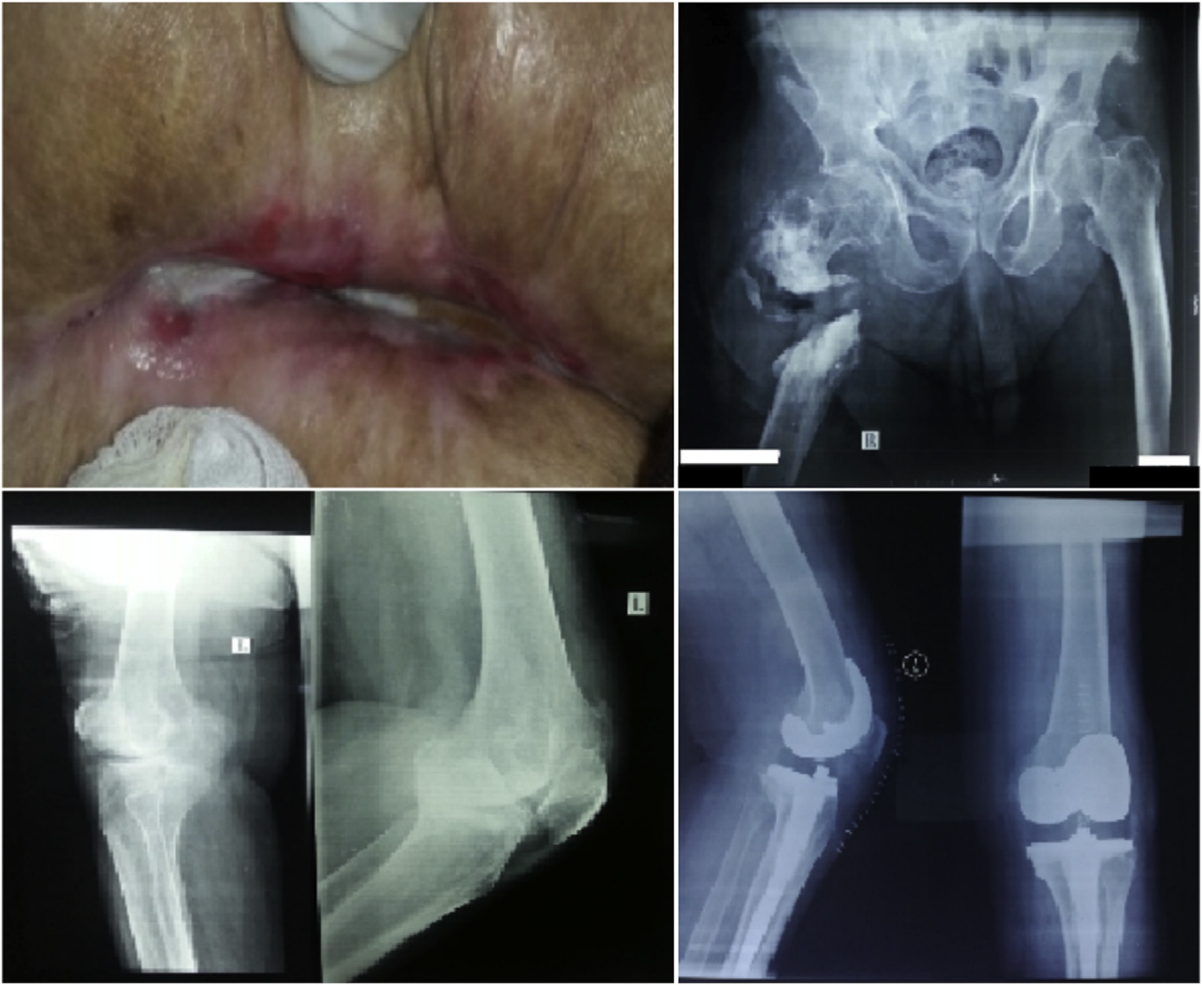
Clinical photo and X-ray image of the infected right femur, and preoperative and postoperative X-ray images of the left knee.
